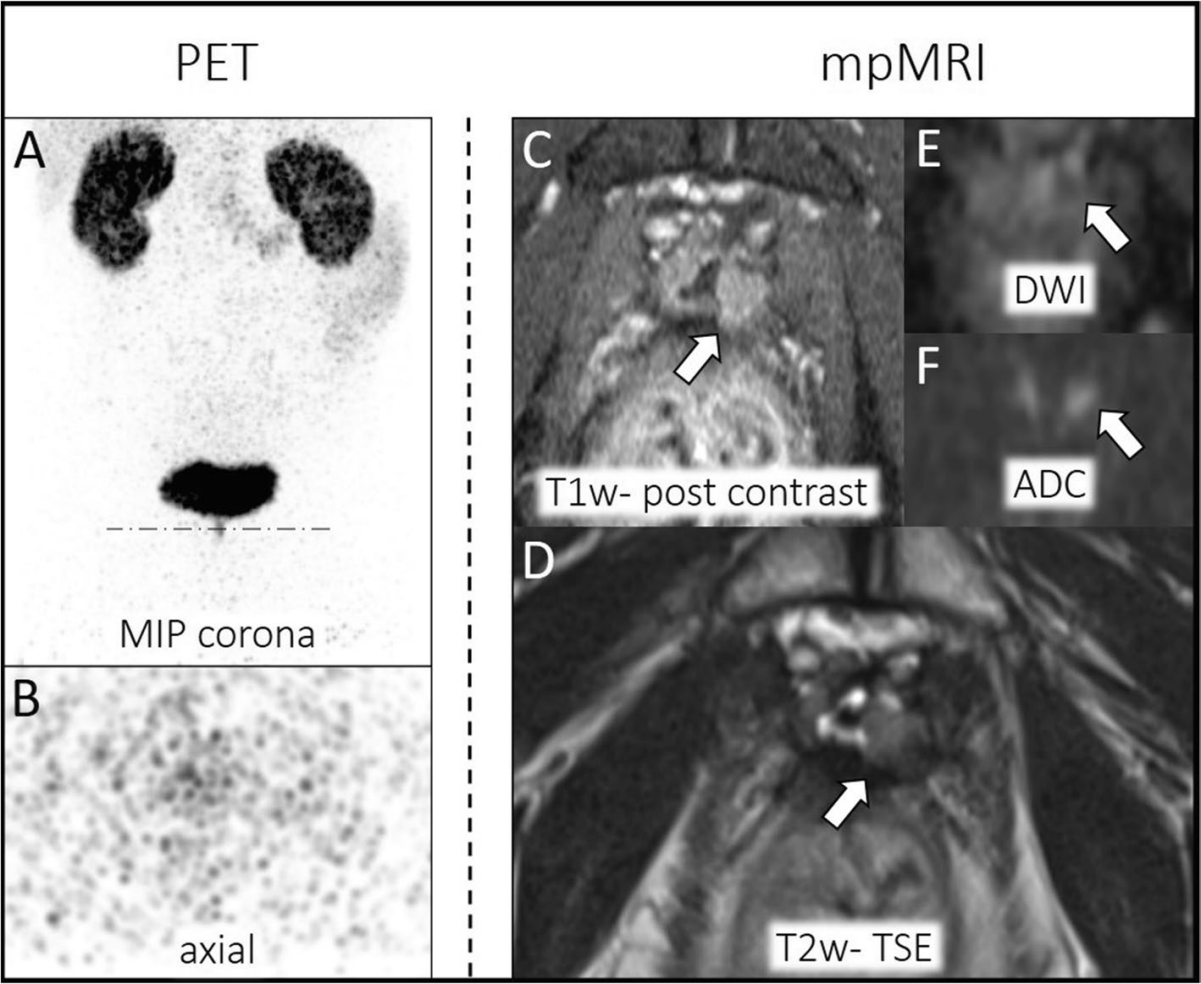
Local recurrence of PCa arising from the vesico-urinary anastomosis. No suspicious uptake is visible in the ^68^Ga-PSMA-11 PET component (left A-B: MIP corona, axial dataset) due to tracer accumulation in the bladder. Dashed line in MIP corona (A) indicates axial PET layer (B). MpMRI depicts tumor relapse (right C-F, white arrow: T1w post contrast, T2w-TSE; DWI, ADC)
